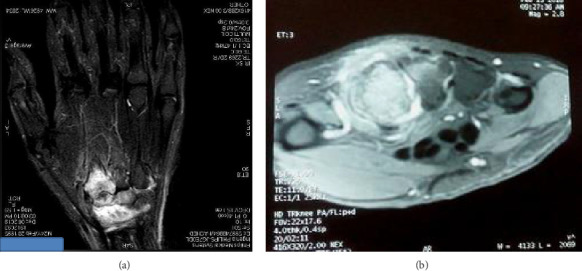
Magnetic resonance imaging (MRI) showing bone marrow oedema in the trapezoid and the second metacarpal bones, synovial effusion, and tissue oedema around the tumor: (a) coronal T2-weighted fat-saturated MRI sequences and (b) transverse T1-weighted gadolinium-enhanced MRI sequences.
